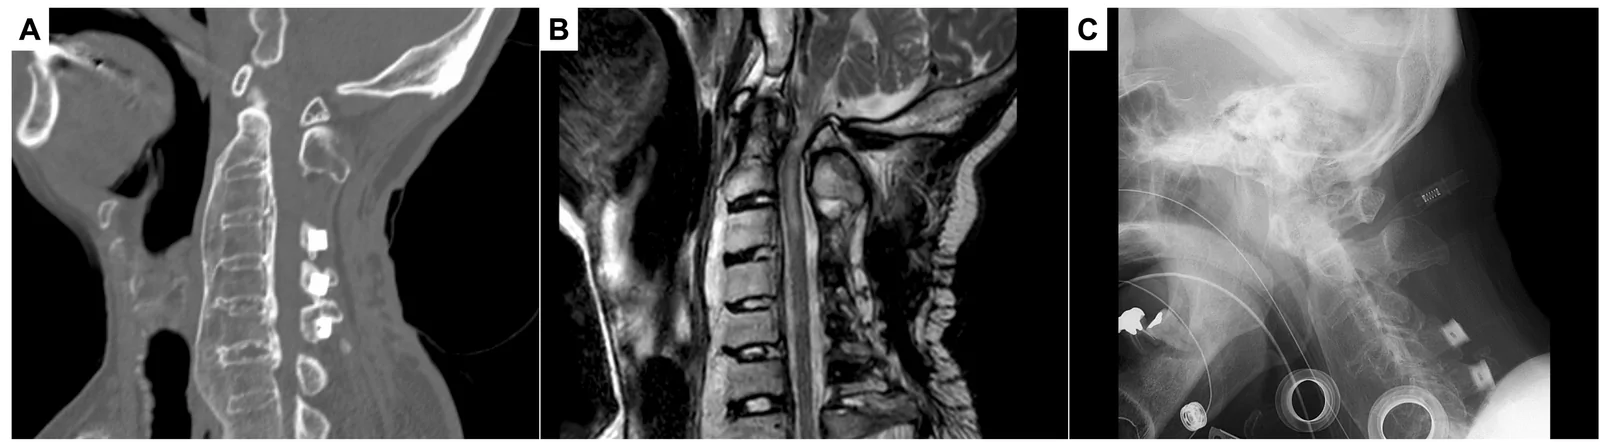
Preoperative CT, MRI, and dynamic imaging of the cervical spine. Mid-sagittal CT (A) demonstrates continuous OALL and OPLL from C2 to T1 without apparent acute injuries such as fracture, disc injury, or posterior element injury. Mid-sagittal T2-weighted MRI (B) shows canal stenosis due to a retro-odontoid pseudotumor accompanied by intramedullary signal change from C1 to C3. Dynamic imaging in neck flexion (C) reveals an ADI of 5.6 mm and a SAC of 15.5 mm. Abbreviations: ADI, atlas-dens interval; CT, computed tomography; MRI, magnetic resonance imaging; OALL, ossification of the anterior longitudinal ligament; OPLL, ossification of the posterior longitudinal ligament; SAC, space available for the spinal cord.
